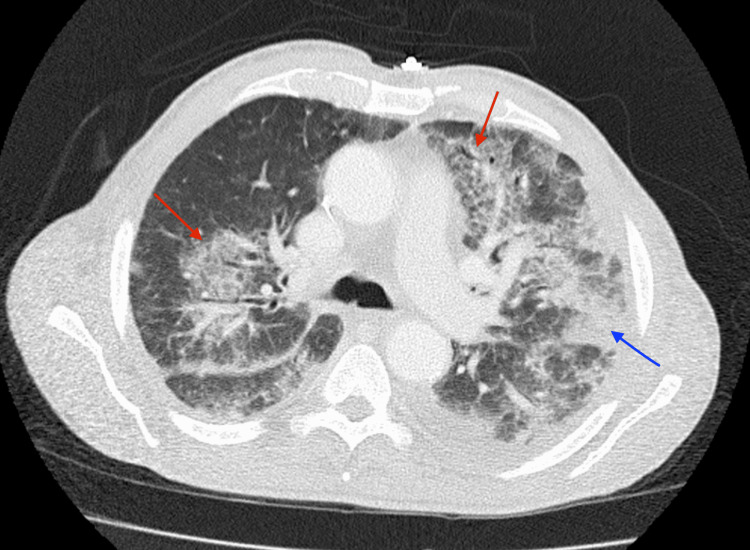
Computed tomography of the chest on day four of azacitidine Red arrows indicate ground-glass opacities, blue arrow indicates alveolar opacities.
